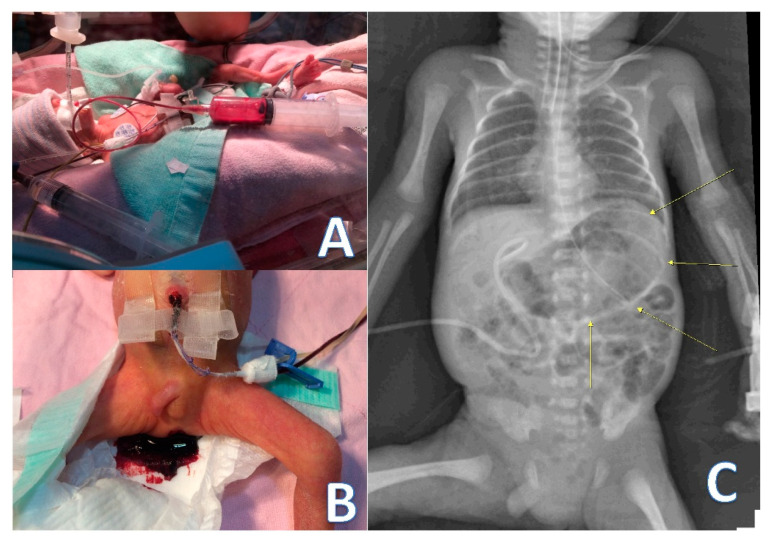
Clinical presentations and distended gastric bubble on an abdominal X-ray. (A) Massive blood drainage from the oral gastric tube, (B) dark red blood passage noted on the diaper, and (C) a small heart and the extensively distended gastric bubble occupied by blood (indicated by the yellow arrows).
